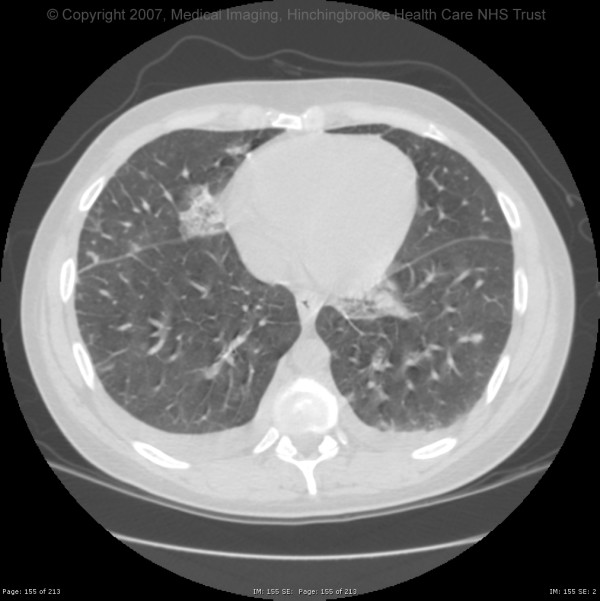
Computed tomography of the chest showing multiple cavitating lung lesions.
